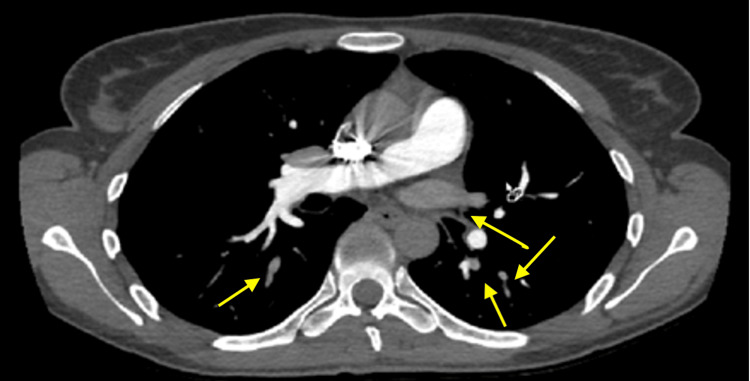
Helical computed tomography pulmonary angiography demonstrating the resolution of embolism in the right pulmonary artery
